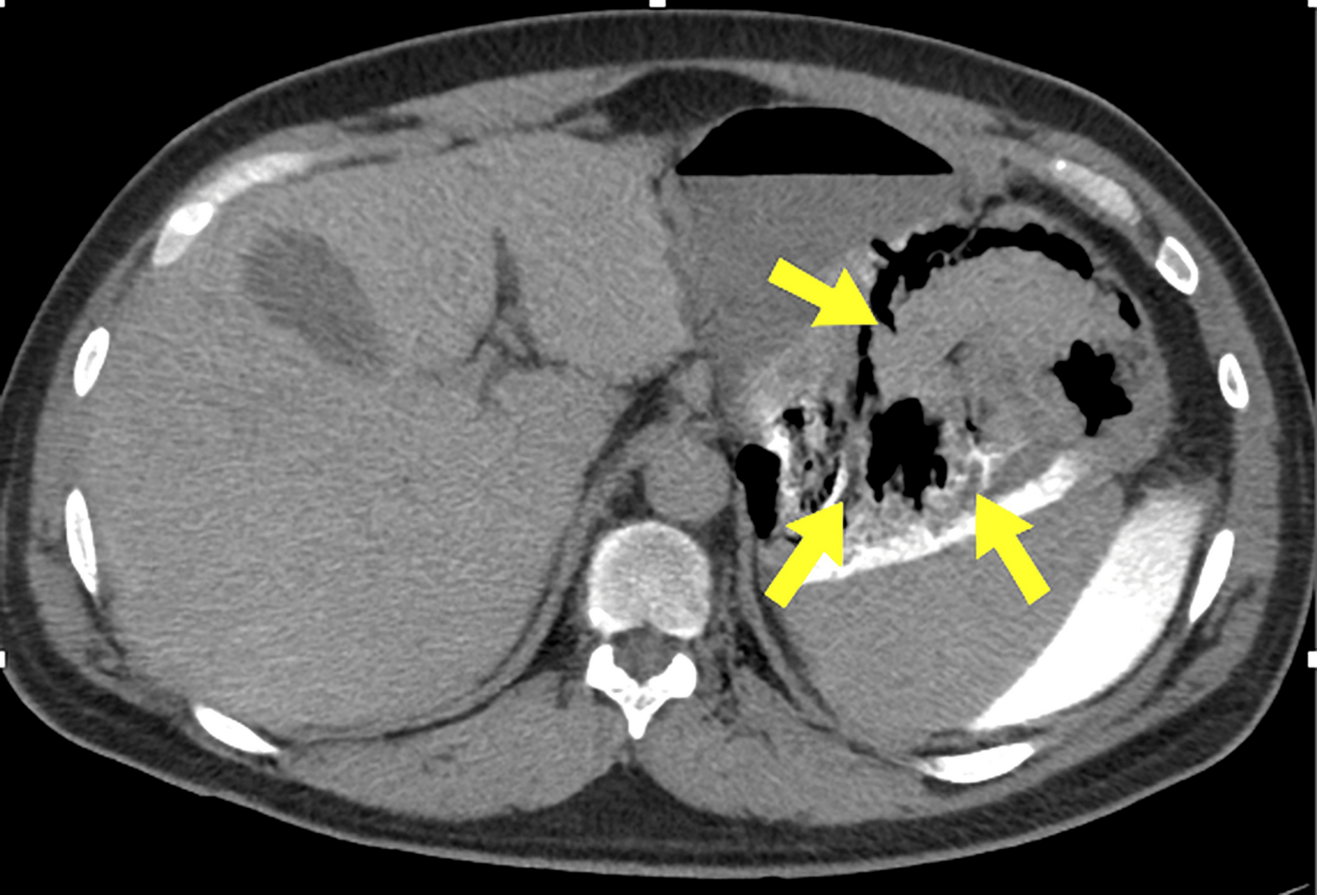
Contrast-enhanced CT findings of late gastrointestinal necrosis Axial contrast-enhanced CT of the abdomen demonstrating non-enhancing thickening of the posterior gastric wall and the adjacent duodenal wall (yellow arrows), associated with intramural gas and surrounding inflammatory infiltration, consistent with ischemic and infected necrosis as a late complication of severe acute pancreatitis. CT: computed tomography
